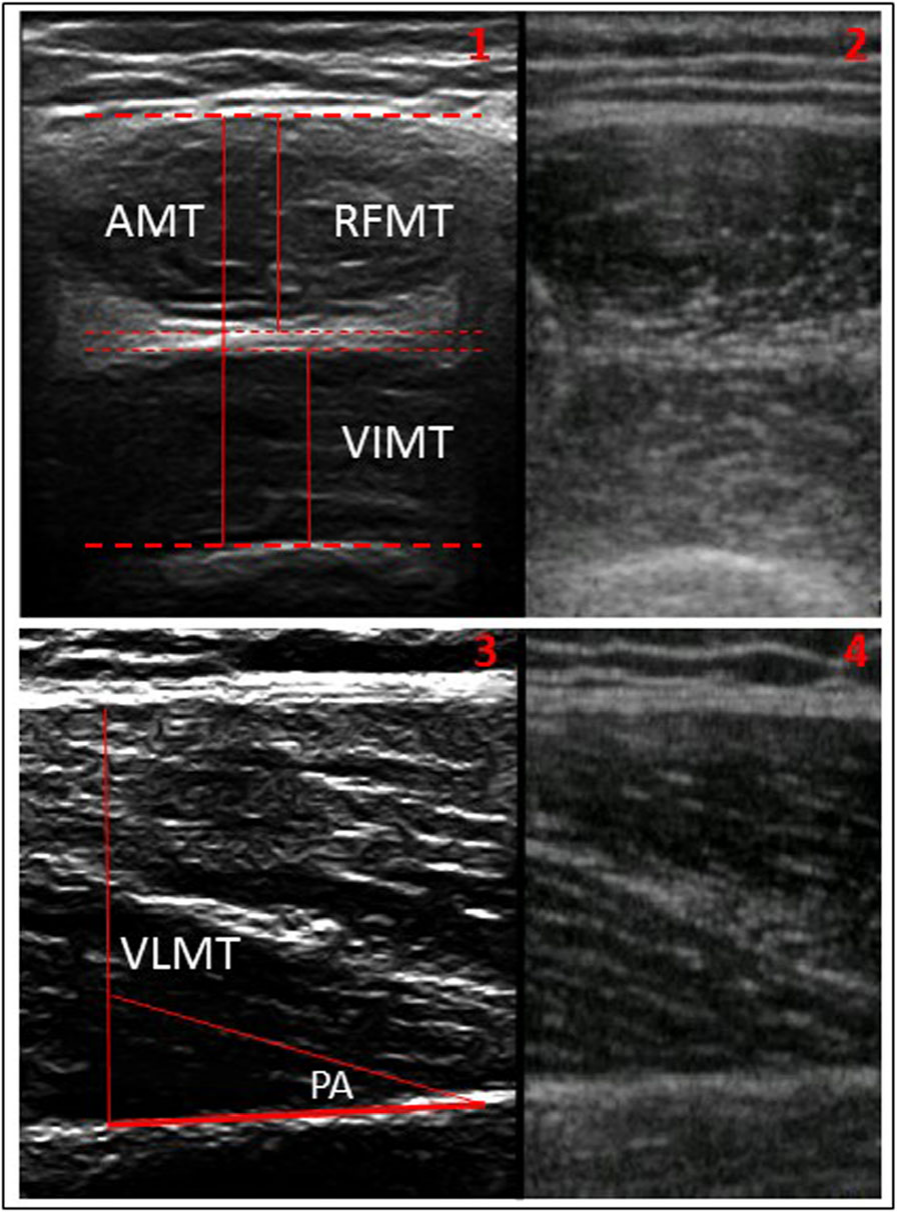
Representative ultrasound scans of the anterior thigh from the Telemed and Vscan systems (panels 1 and 2 respectively) and vastus lateralis (panels 3 and 4 respectively) Legend: AMT: anterior thigh muscle thickness, RFMT: rectus femoris muscle thickness, VIMT: vastest intermedius muscle thickness, VLMT: vastus lateralis muscle thicknes, PA: pennation angle.
